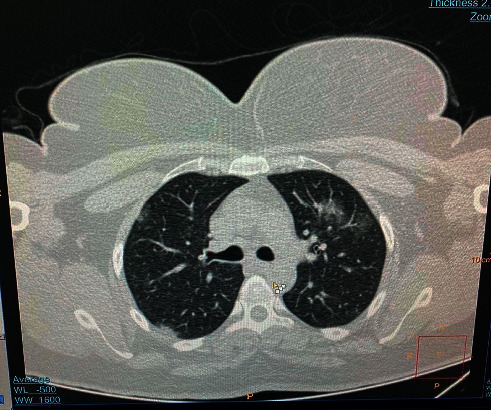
Patchy ground-glass and peripheral infiltration in chest CT scan.
